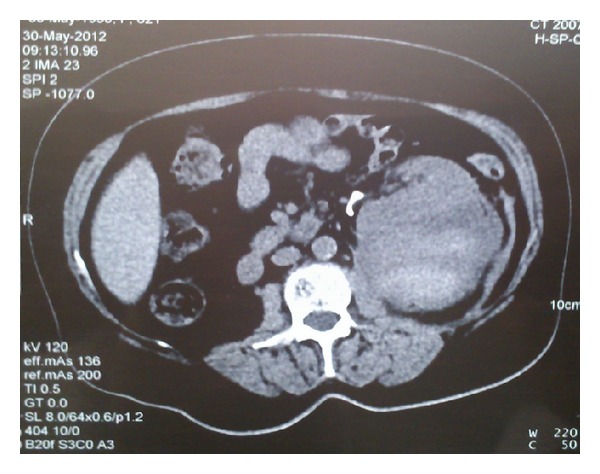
CT of the abdomen 3 weeks after stent placement showing stabilization of the perirenal hematoma with the D-J stent repositioned in the renal pelvis.
